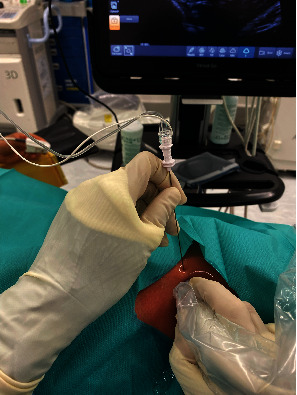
Insertion of needle under ultrasonic guidance.
